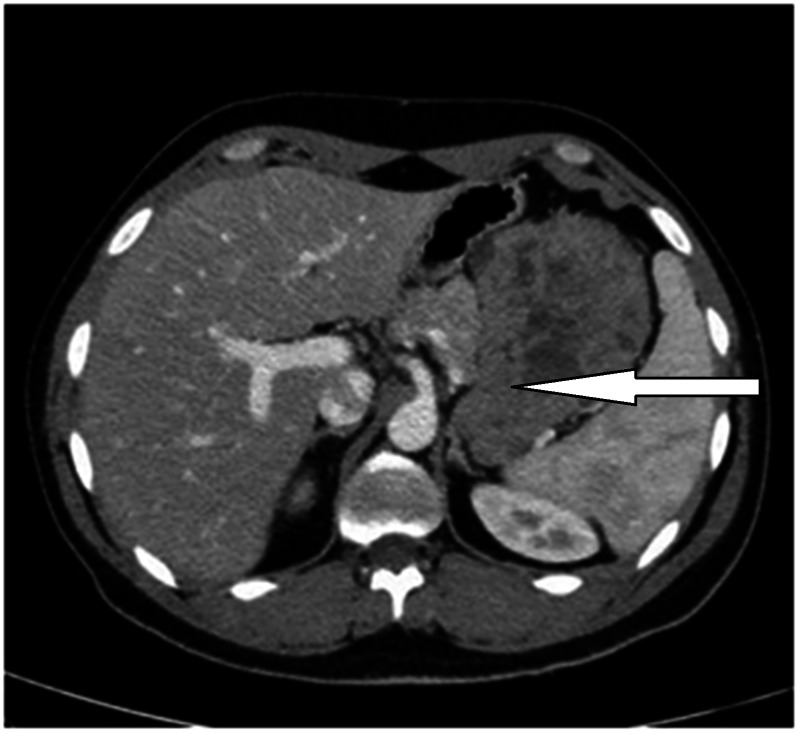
CT scan image (axial view) showing the mass with areas of cystic necrosis, reaching the left adrenal gland and the medial surface of the spleen with no evidences of invasion.
